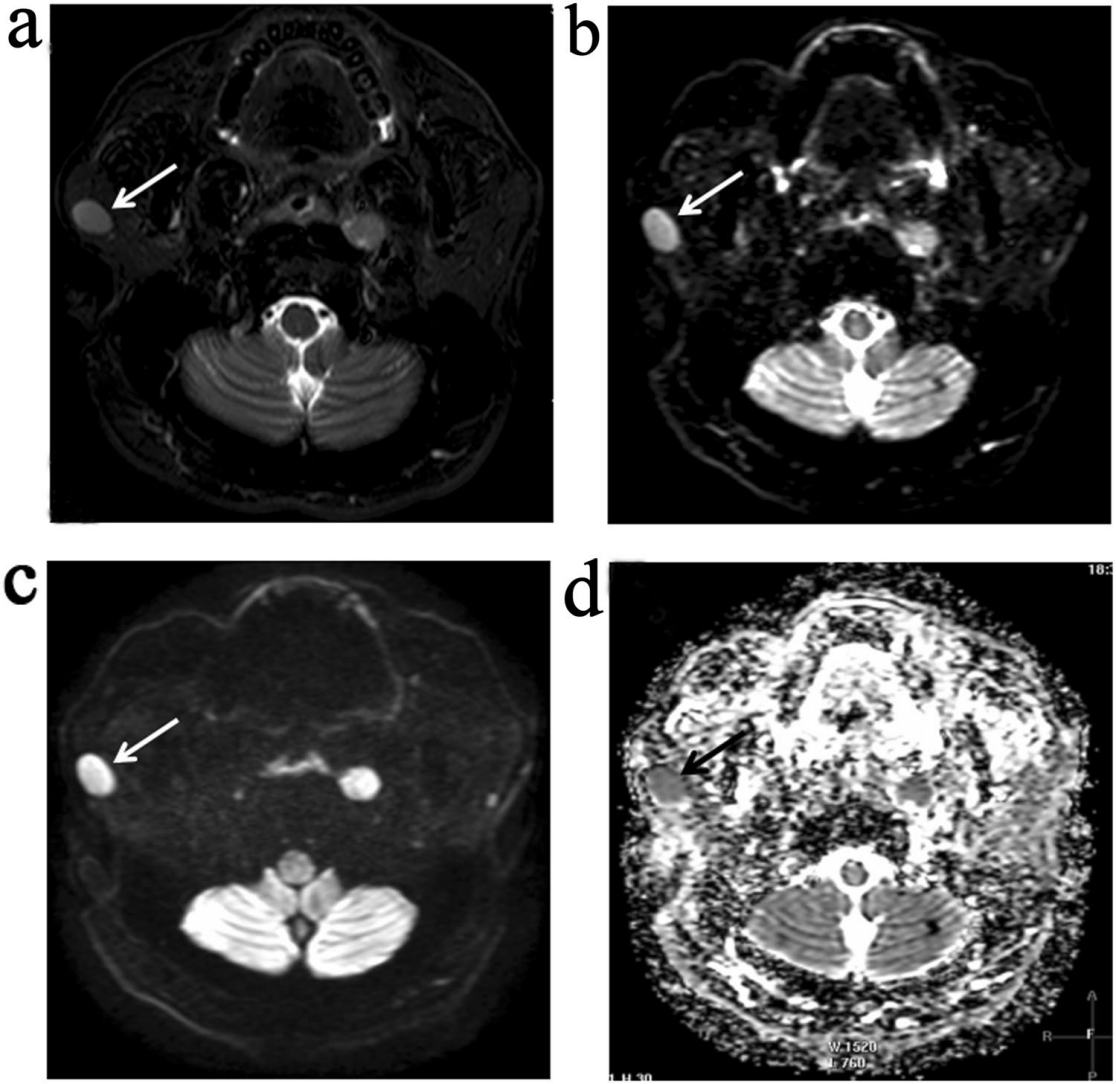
MR images of a metastatic PLN without necrosis (arrows) showing the homogenous signal intensity on a transverse T2-weighted image (a). Axial diffusion-weighted MR images show high signals achieved at both *b* = 0 s/mm^2^ (b) and *b* = 800 s/mm^2^ (c). Consequently, they show low intensity on the ADC map (d).
